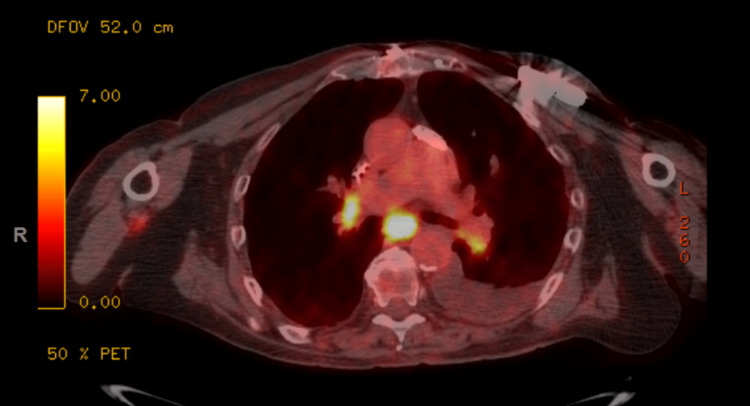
FDG-avid lymphadenopathy in the mediastinum FDG: 18F-fluorodeoxyglucose
